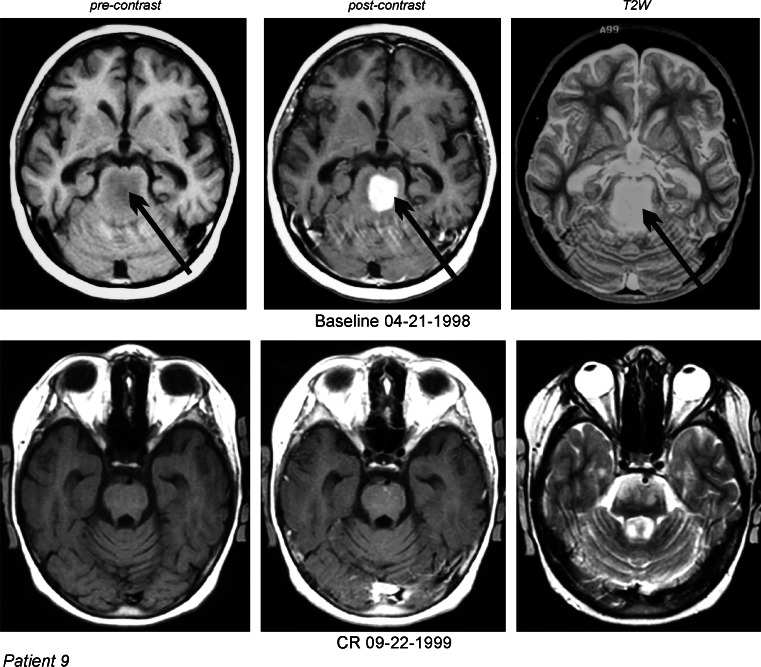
DIPG in a 7-year-old female (case 9) which recurred after radiation therapy and chemotherapy with etoposide. MRI of the head: 1—T1 nonenhanced, 2—contrast-enhanced, and 3—T2W images. MRI documented PR. *Arrows* indicate tumors
